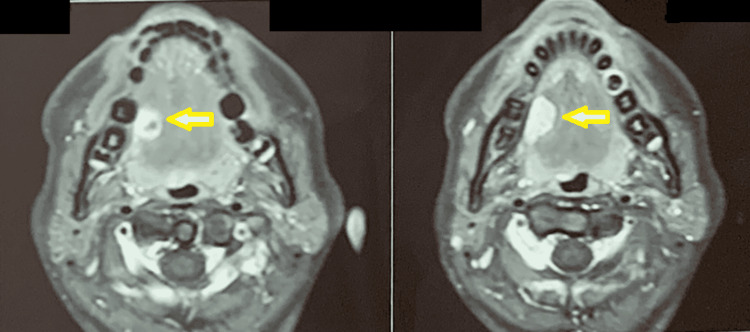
MRI of the neck impression revealing a mildly enhancing, fairly defined, diffusion-restricting, T2/STIR hyperintense lesion with internal hypointensity in the right lateral aspect of the tongue (arrow)
